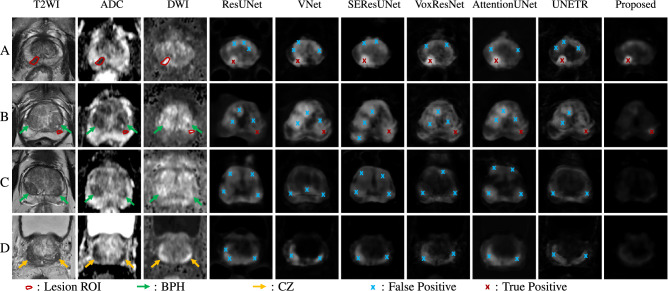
Visualizations of csPCa detection results among different models ResUNet^43^, VNet^44^, SEResUNet^25^, VoxResNet^46^, AttentionUNet^45^, UNETR^47^ on two patients with csPCa (A and B) and two without csPCa (C and D). From left to right, the first column shows the T2WI images, the second column shows the ADC images, the third column shows the high-B DWI images and all other columns show the detection probability maps generated by different models for each patient A, B, C and D correspondingly. Red contours shown on T2WI, ADC, and high-B DWI images indicate the contour of csPCa lesions. Yellow arrows point to the regions of CZ, and Green arrows point to the regions of BPH on the MR images. Blue crossings represent FP predictions, and red crossings represent TP predictions.
